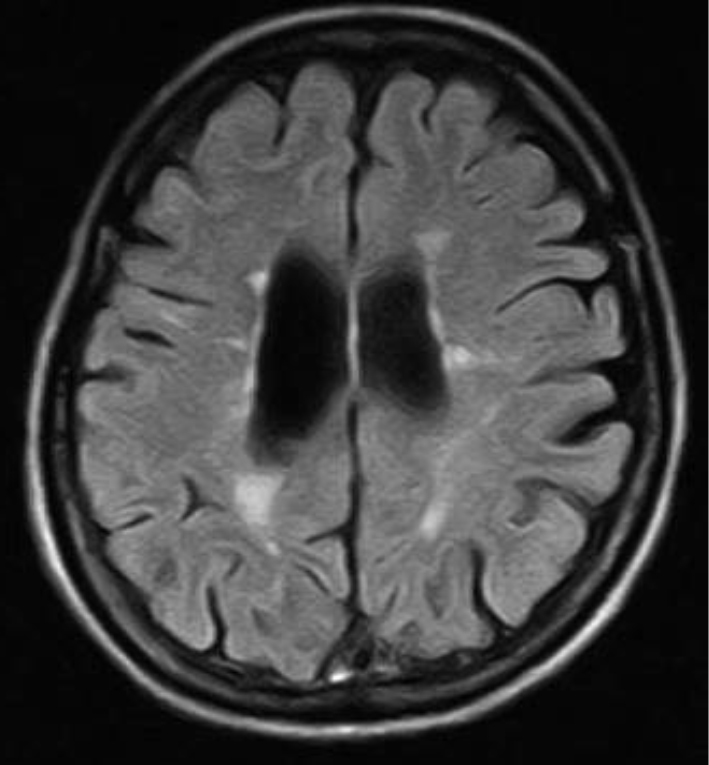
Brain MRI (fluid-attenuated inversion recovery (FLAIR)) showed multiple hyperintense white matter lesions adjacent to the bilateral ventricles at 44 years of age.
